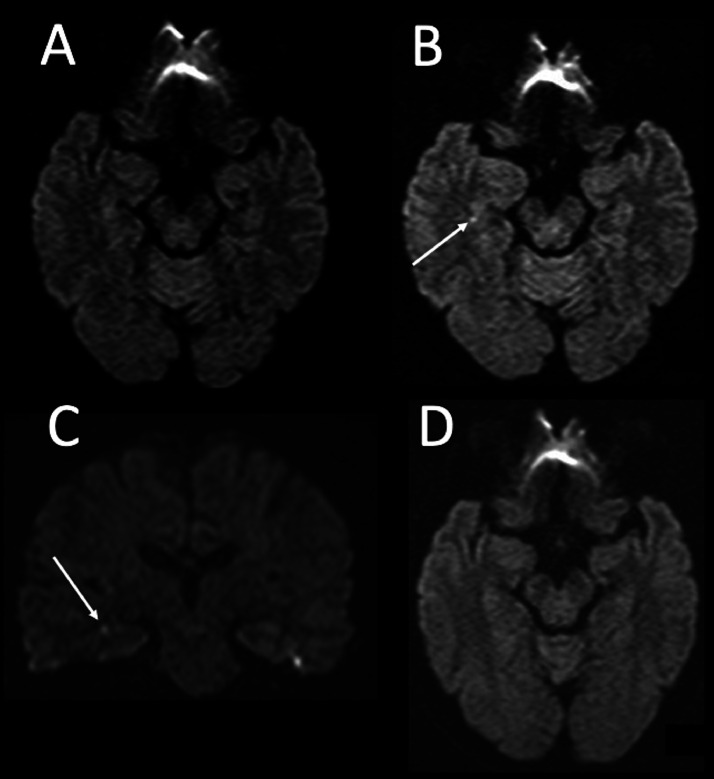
Time course of hippocampal microinfarction on diffusion weighted imaging. (A) Axial diffusion-weighted imaging (DWI) obtained 3 hours post-onset, showing no significant signal changes. (B, C) Axial and coronal DWI, respectively, obtained 2 days post-onset, both demonstrating a punctate high signal in the right hippocampus (arrow). (D) Axial DWI obtained 9 days after onset, showing disappearance of the previously observed abnormal signal in the right hippocampus.
